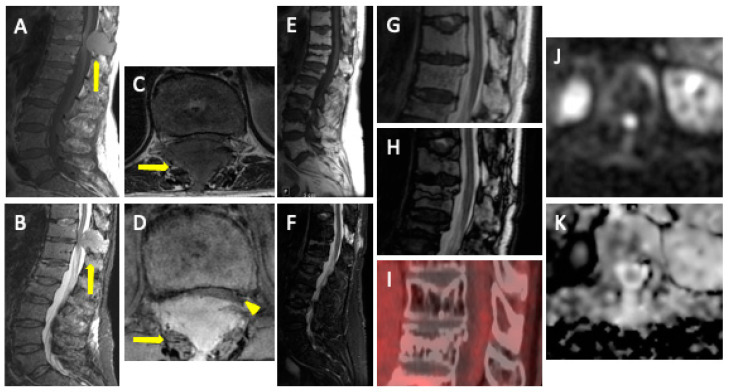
MM with associated plasmacytoma. T1WI (A,C) and STIR (B,D) show diffuse involvement of the bone marrow with soft tissue expansive mass (plasmacytoma) in the posterior elements of T11 (arrows) with severe stenosis of the vertebral canal (arrowhead in (D)). T1 (E) and STIR (F) show successful post-treatment changes with fat replacement (hyperintensity in T1) and >20% signal drop between in-phase (G) and opposed phase (H). There is an absence of FDG uptake (I) and a high SI on *b*-900 DWI (J), with an increase in ADC (K) (“T2-shine-through” effect), which demonstrates a lack of relapse.
